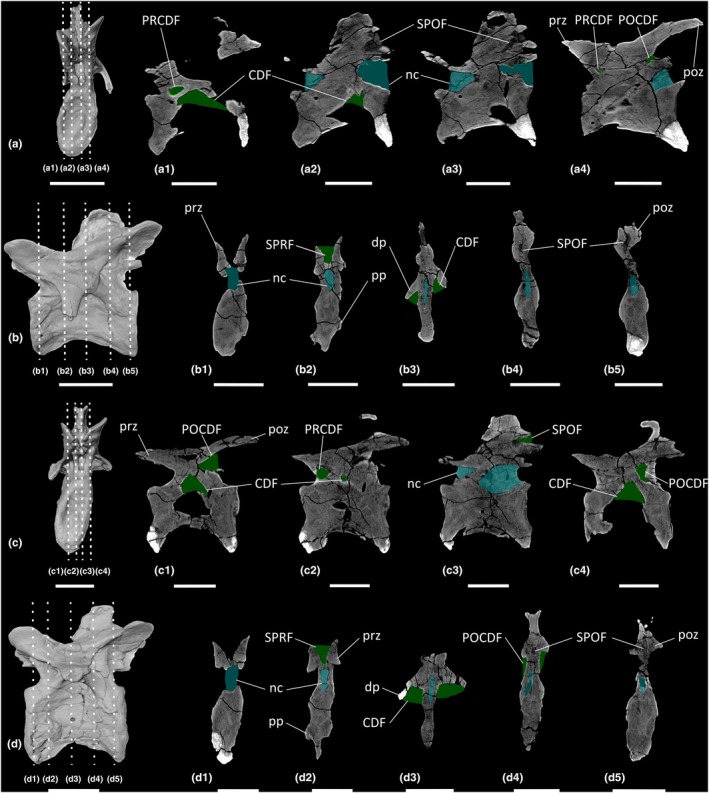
CT scan data of *Plateosaurus longiceps* MB.R.4430 ‘Skeleton C’ cervical vertebrae. (a, b) Cervical vertebra 9 MB.R.4430.20, (c, d) cervical vertebra 10 MB.R.4430.21; (a, c) surface scan anterior view, (b, d) surface scan lateral view; (a1–a4, c1–c4) CT scan slices in parasagittal cross‐section, (b1–b5, d1–d5) CT scan slices in transverse cross‐section. CDF, centrodiapophyseal fossa; dp, diapophysis; nc, neural canal; POCDF, postzygapophyseal centrodiapophyseal fossa; poz, postzygapophysis; pp, parapophysis; PRCDF, prezygapophyseal centrodiapophyseal fossa; prz, prezygapophysis; SPOF, spinopostzygapophyseal fossa; SPRF, spinoprezygapophyseal fossa. Key: Blue highlight, neural canal; green highlight, fossa. Scale bars = 5 cm.
